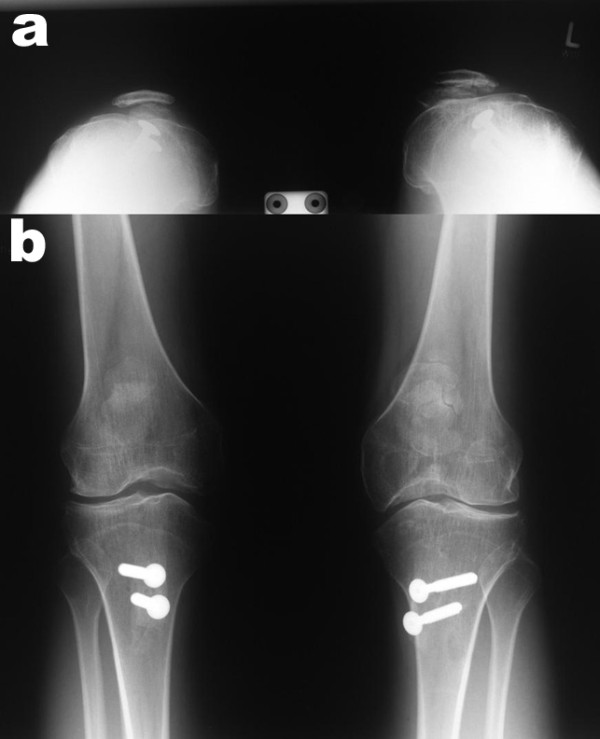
Postoperative x-ray (a: skyline view (60° flexion) and b: anterior posterior view) of both knees demonstrating well centred patellae.
